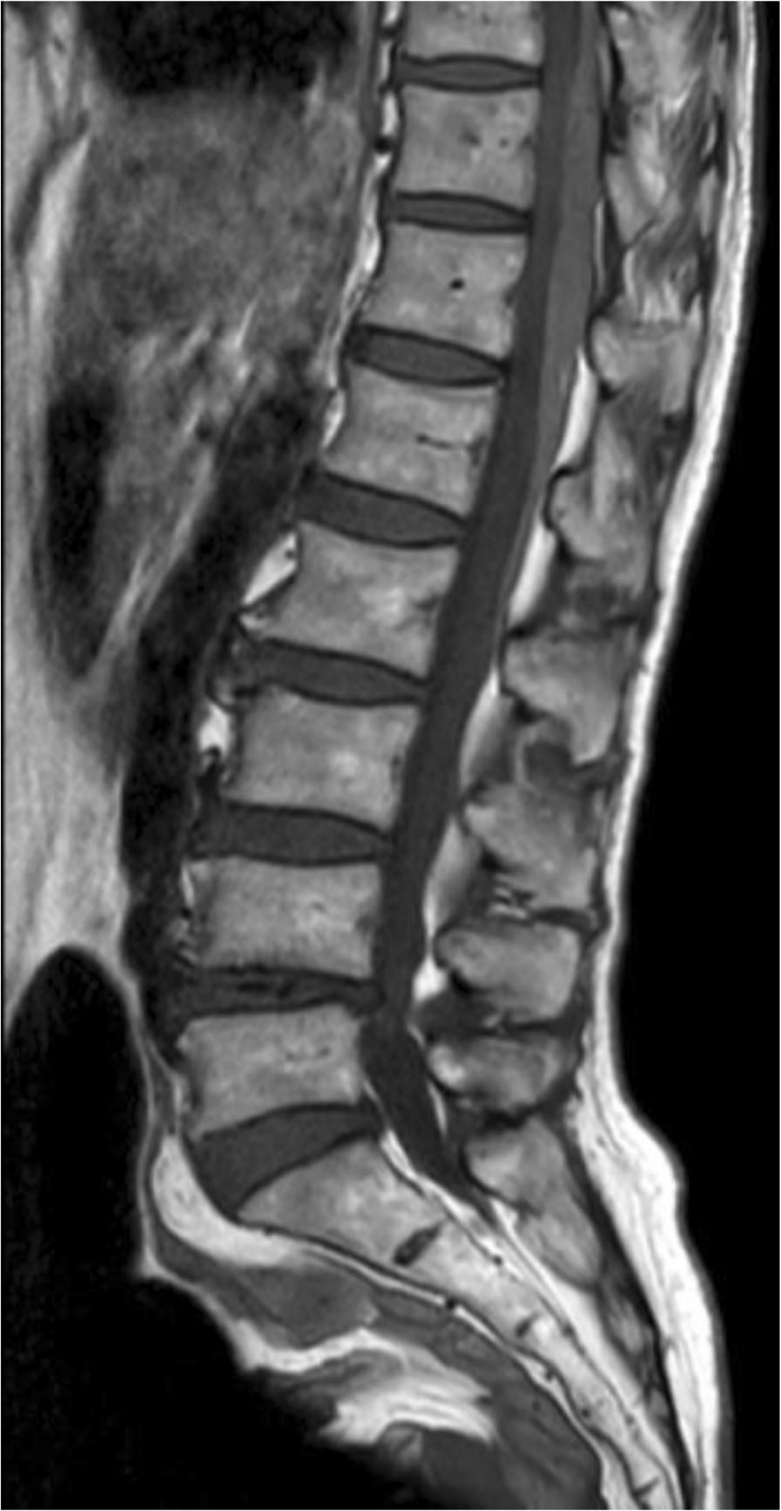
T1-weighted image of the patient’s spine. T1-weighted magnetic resonance image showing compression of the posterior aspect of the spinal cord by a hematoma extending from T10–L1
